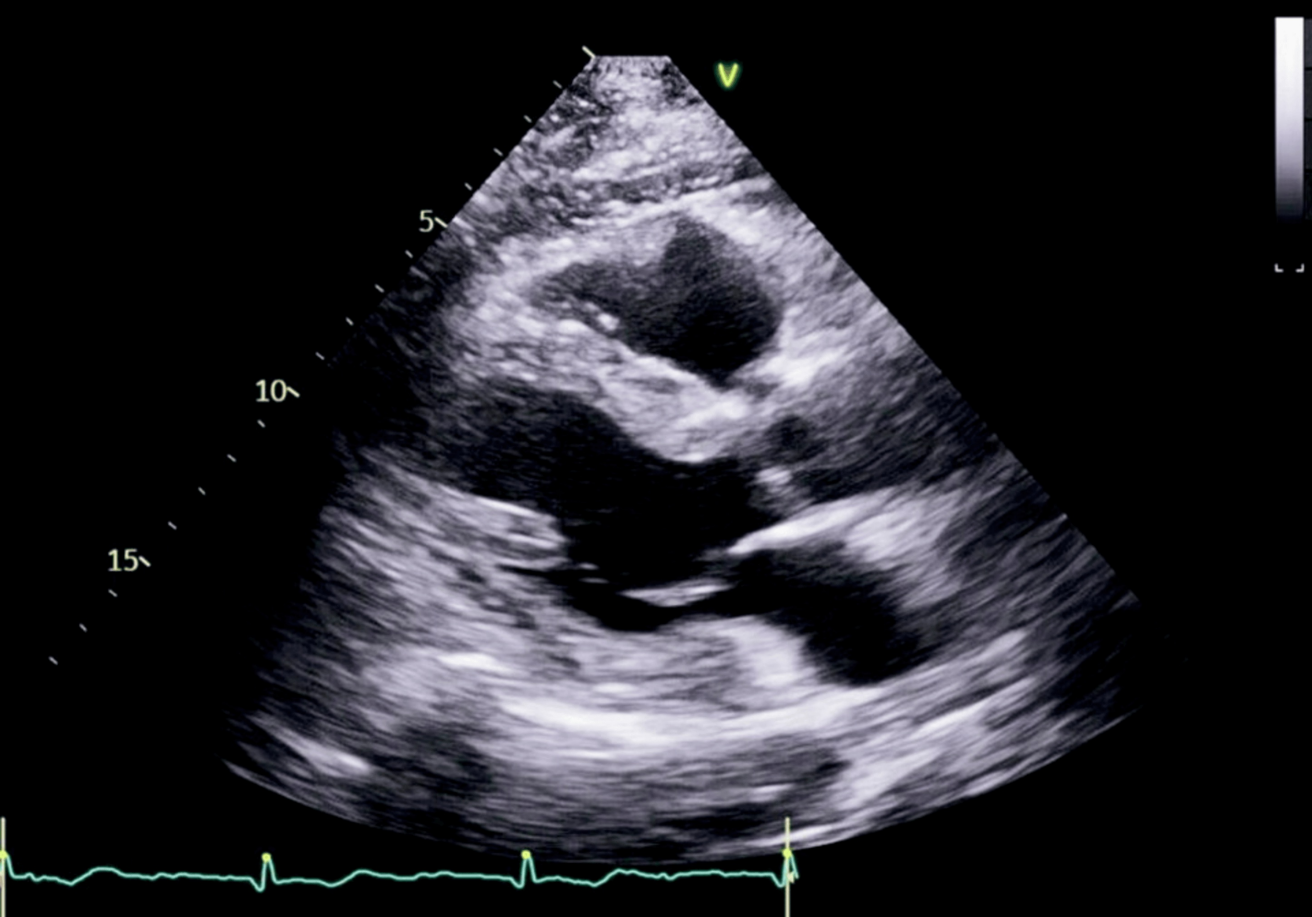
Parasternal long-axis view acquired via transthoracic echocardiography, visualizing the patient's cardiac anatomy
